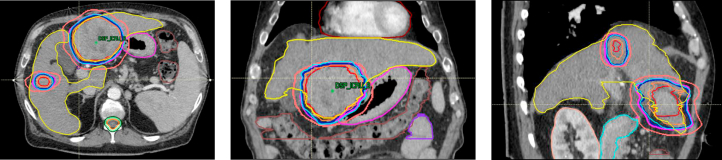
Planning CT in arterial phase (axial, coronal, and sagittal cuts) showing SBRT plan for HCC – 3 lesions treated with a dose of 27.5 Gy in 5 fractions. 60-year-old gentleman diagnosed with multifocal HCC, BCLC B with Child-Pugh B8 with 3 lesions treated with SBRT as a bridge to transplant. Red- Gross tumour volume, Cyan- Planning target volume, Blue: 100% isodose line, Pink: 95% isodose line and Orange:70% isodose line.
